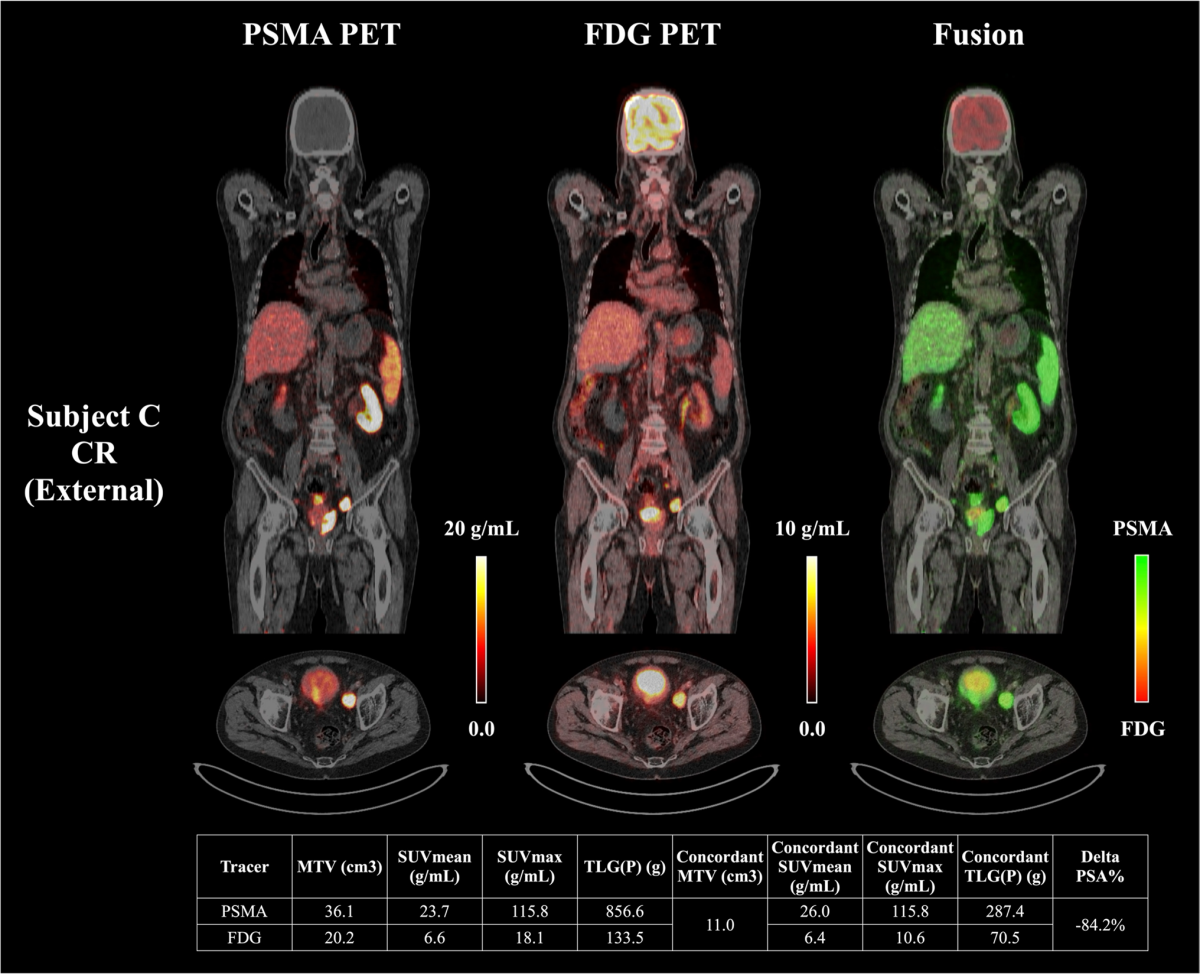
Representative case from the external Augsburg cohort demonstrating complete response, characterized by high PSMA uptake in concordant lesions. This patient exhibited complete response (CR) following PSMA-RLT, with a PSA decline of 84.2%. The SUV_mean_[PSMA Concordant] in this patient was 23.7 g/mL, exceeding the identified cutoff value (12.1 g/mL)
